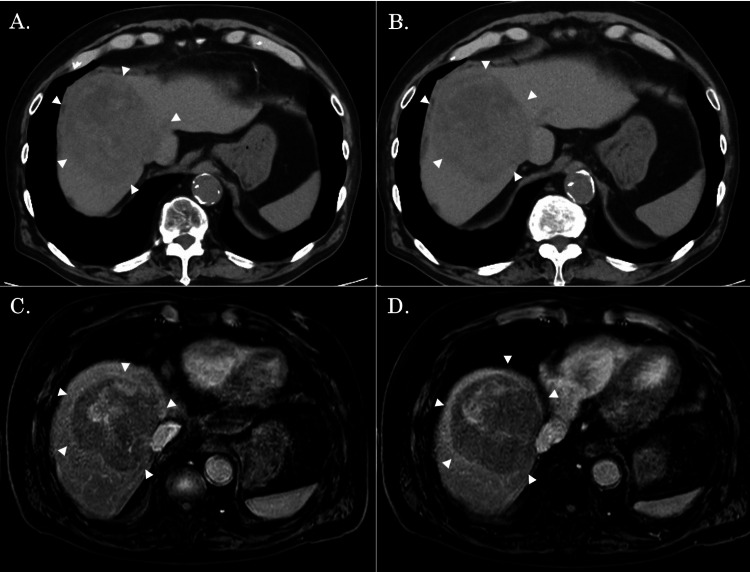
CT and MRI findings before the chemotherapy. (A, B) An enhanced CT scan showed that the tumor occupied a large space in the right lobe of the liver and was 82 × 78 mm in size (white arrows). (C, D) An MRI showed that the tumor was in contact with the roots of the inferior vena cava and the right and middle hepatic veins (white arrows).
